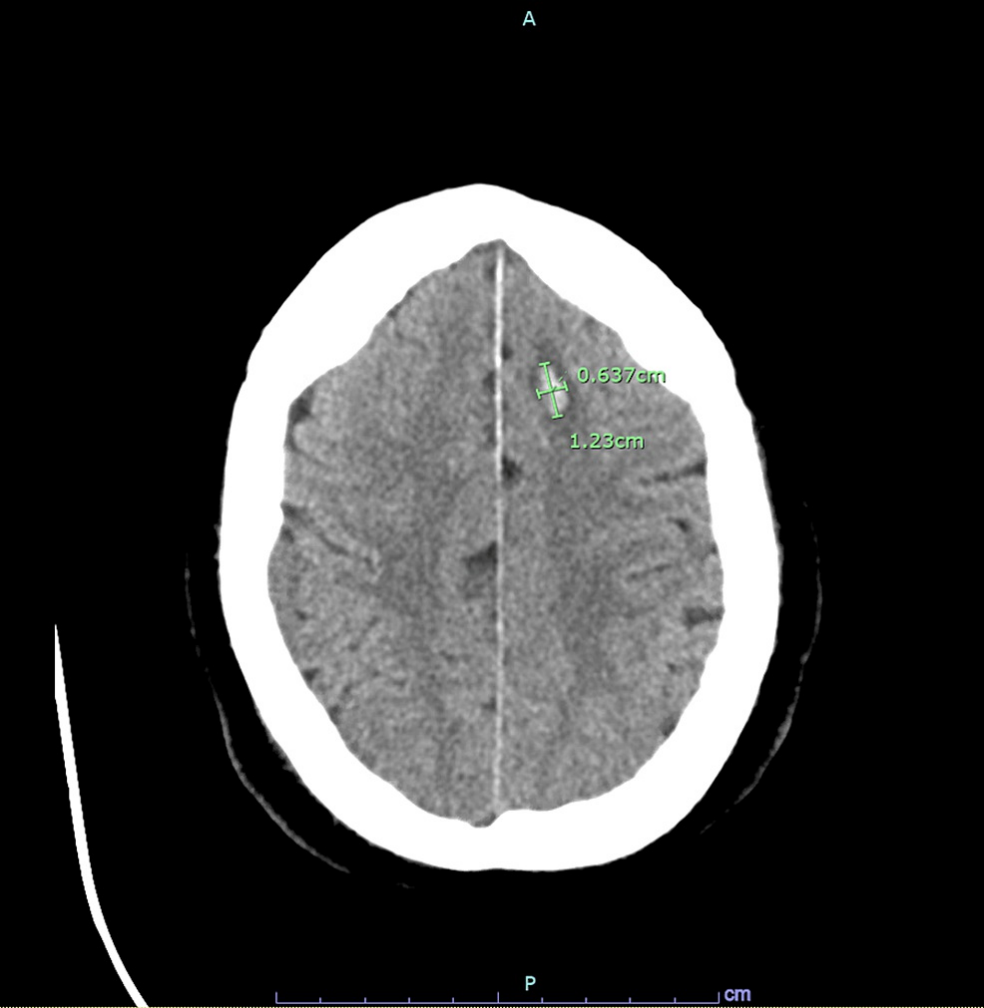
CT of the head without contrast. Small left frontal parenchymal hematoma measuring 1.23 × 0.637 cm in transaxial dimensions.
